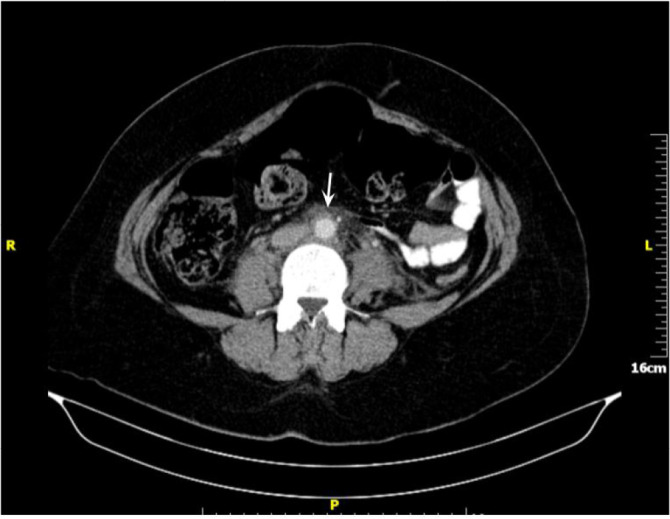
CT findings in periaortitis, low-density soft tissue around the abdominal aorta.
